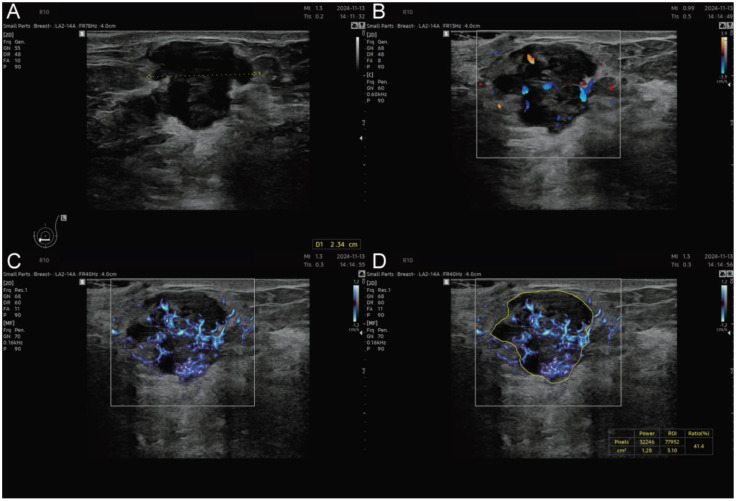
Grayscale ultrasound, CDFI, and MV-Flow images of invasive breast carcinoma in the left breast of a 56-year-old female. (A) Grayscale ultrasound features: irregular morphology, ill-defined margins, heterogeneous internal echogenicity, posterior acoustic enhancement, and anterior protrusion into the subcutaneous fat layer. (B) CDFI demonstrates Adler grade 3 vascularity. (C) MV-Flow reveals Adler grade 3 vascularity. (D) A quantitative Vascular Index (VI) of 41.4 is obtained in MV-flow mode. The final ultrasound diagnosis was categorized as BI-RADS 4C.
